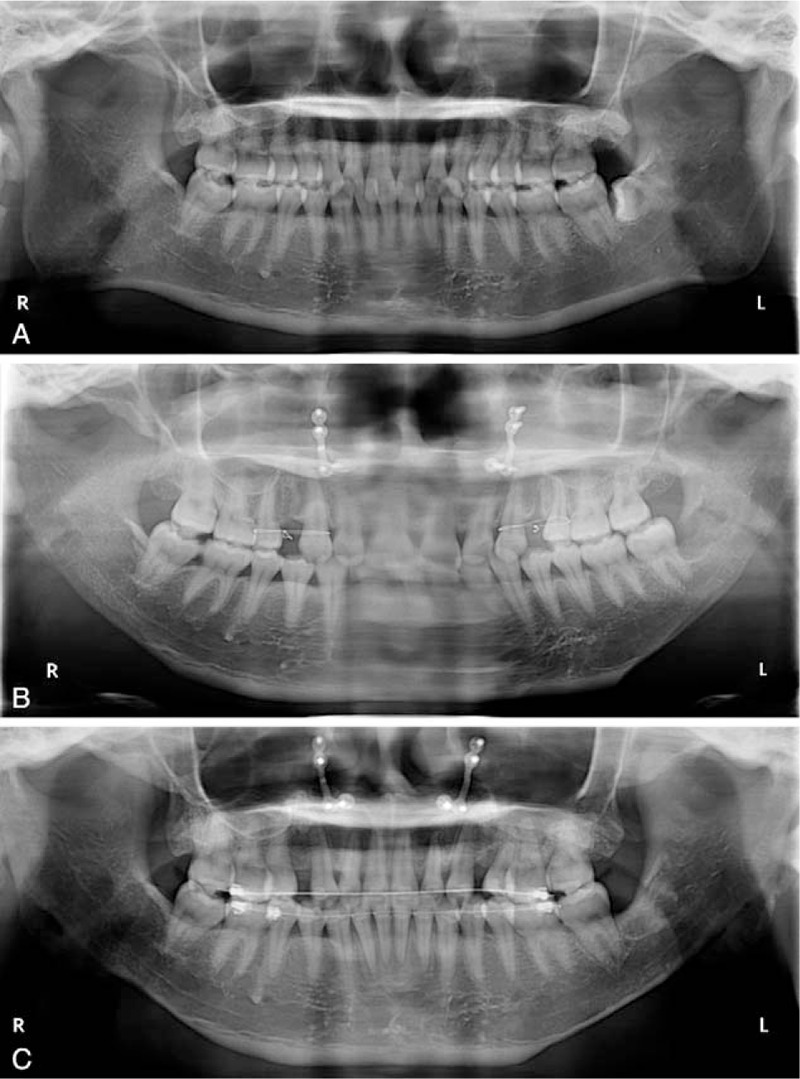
Mandibular panoramic radiographs of patient 3. Before surgery the mandibular rami were bulged with a square angle. The left wisdom tooth was horizontally impacted (A). Mandibular ostectomy was successful. The impacted wisdom tooth was extracted without fracture (B). The long-term post-op radiograph showed complete bone healing (C).
